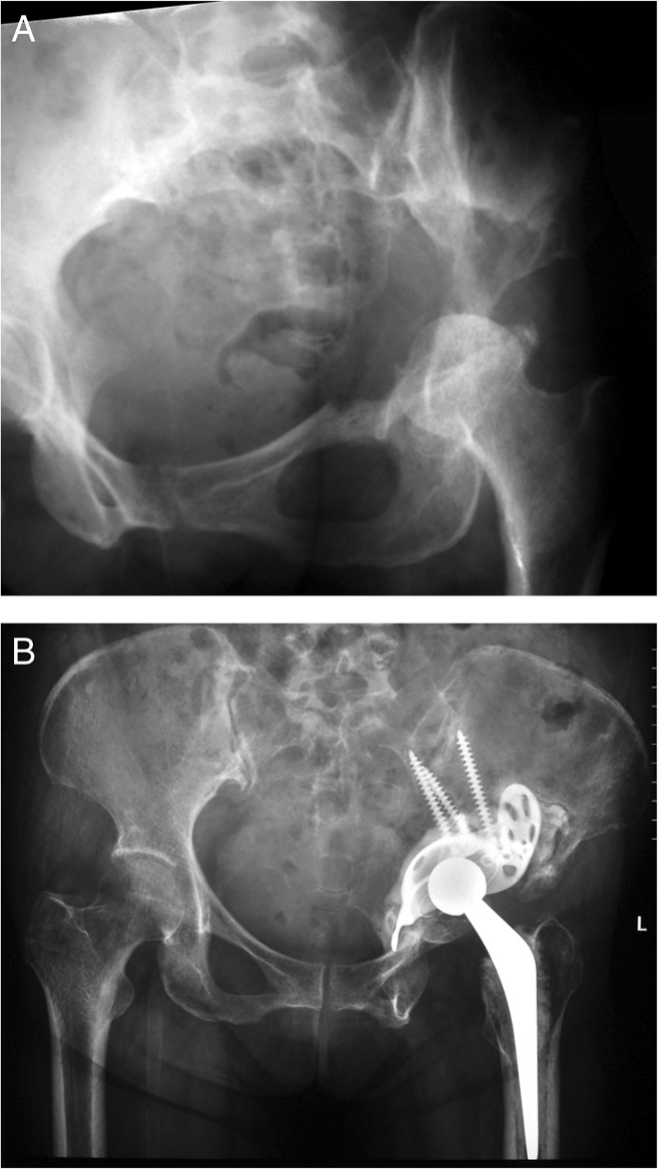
Radiograph of left hip of a 53 year-old woman with class III periacetabular metastatic disease from thyroid carcinoma. (A) preoperative radiograph showed a large lesion involving the superior dome and medial wall. (B) after reconstruction with anti-protusio cage, screws and total hip arthroplasty. The patient was able to walk without walking aid but died of her disease at 28 months after the surgery without any complication.
